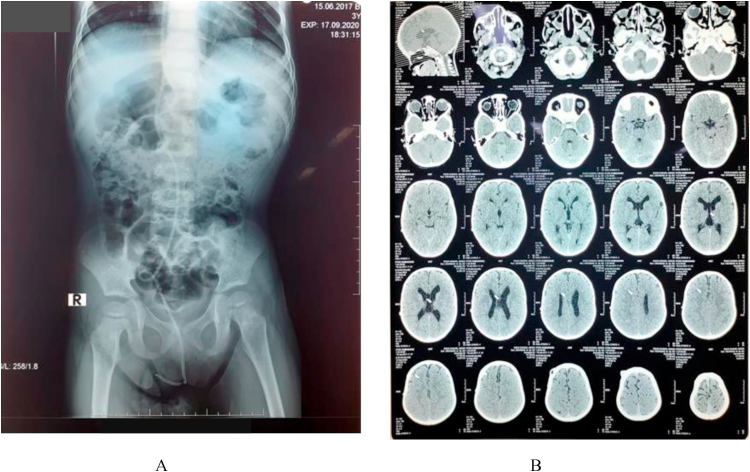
A. Abdominal X-ray, distal peritoneal shunt catheter coiled at lower abdominal quadrant and go off the pelvic area/vagina, B. Head CT-scan revealed mild bilateral ventriculomegaly.
